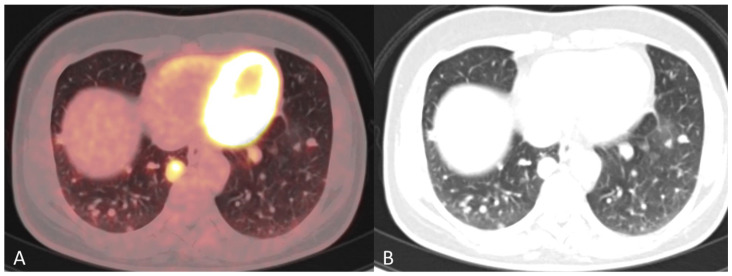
(A) Follow-up axial PET/CT fused and (B) axial CT after three series of chemotherapy shows progression with multiple metastases in both lungs.
